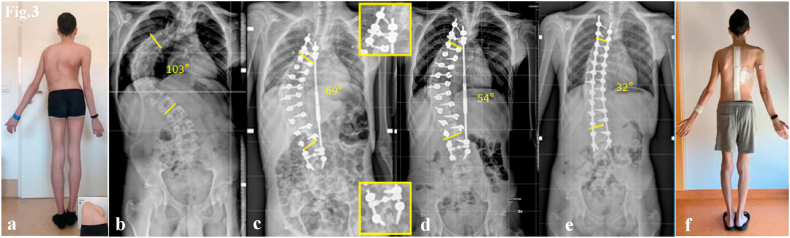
Case 1. 15-year-old male with a Lenke Type 1 curve, treated with T4–L4 posterior spinal fusion. Antero-posterior images show: (a) preoperative clinical assessment with thoracic hump, (b) preoperative radiograph, (c) MCGR implantation prior to distraction and proximal/distal “Sistema Quadro” construct (d) correction after MCGR lengthening, (e) final radiographic correction with definitive rods, (f) final clinical correction.
